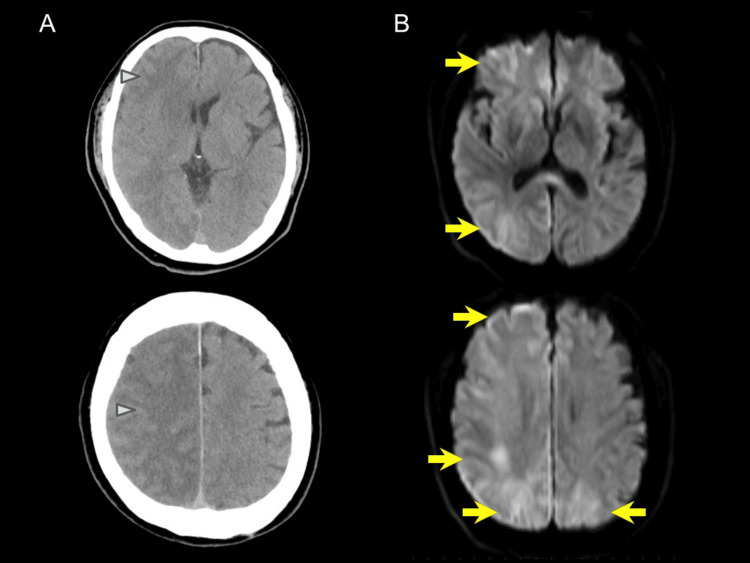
Follow-up brain CT and MRI. Brain CT on Day 6 shows ischemic lesions (arrowheads) and reduction in pneumocephalus (A). Diffusion-weighted MRI on Day 10 reveals multifocal high-intensity areas in the right cerebral hemisphere, left frontal lobe, and occipital lobe (arrows) (B).
